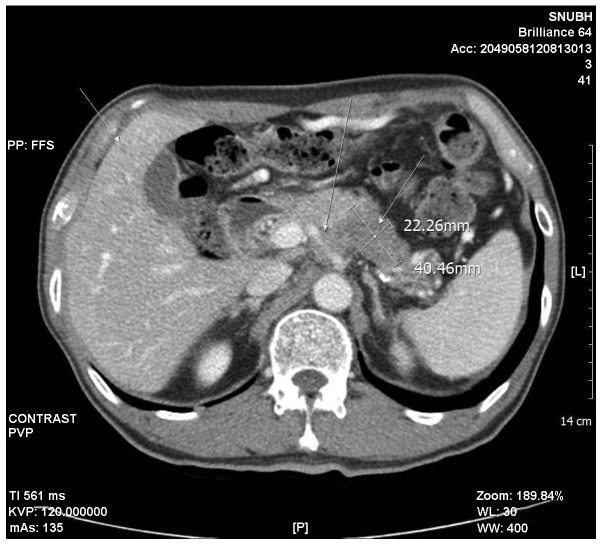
Computed tomography showing a heterogeneous mass in the tail of the pancreas, multiple lymph node enlargements and metastatic nodules in the liver.
